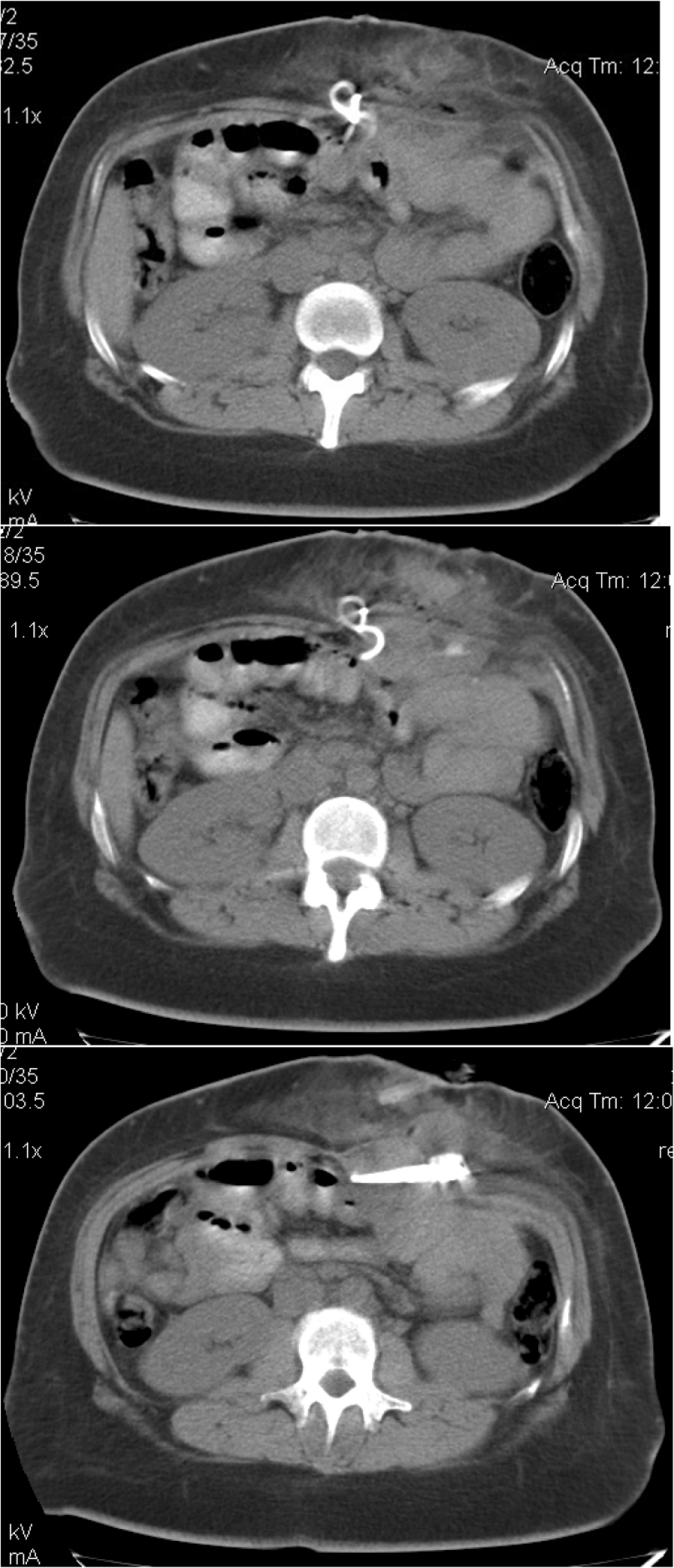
CT of the abdomen and pelvis with oral contrast revealed subcutaneous spherical foreign body (probably the port) with the connected tube extending 10 cm into the abdominal cavity
